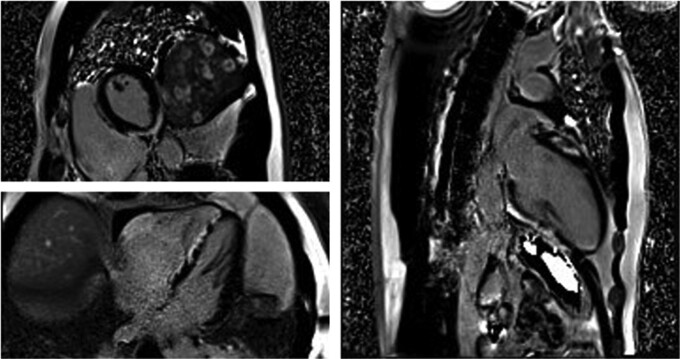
MRI phase contrast inversion recovery images showing multifocal late gadolinium enhancement in the septum and subepicardial and mid-myocardial regions of the basal inferior and anterior walls, consistent with cardiac sarcoidosis. The basal inferior wall also has associated oedema on T2 imaging suggesting ongoing inflammation.
